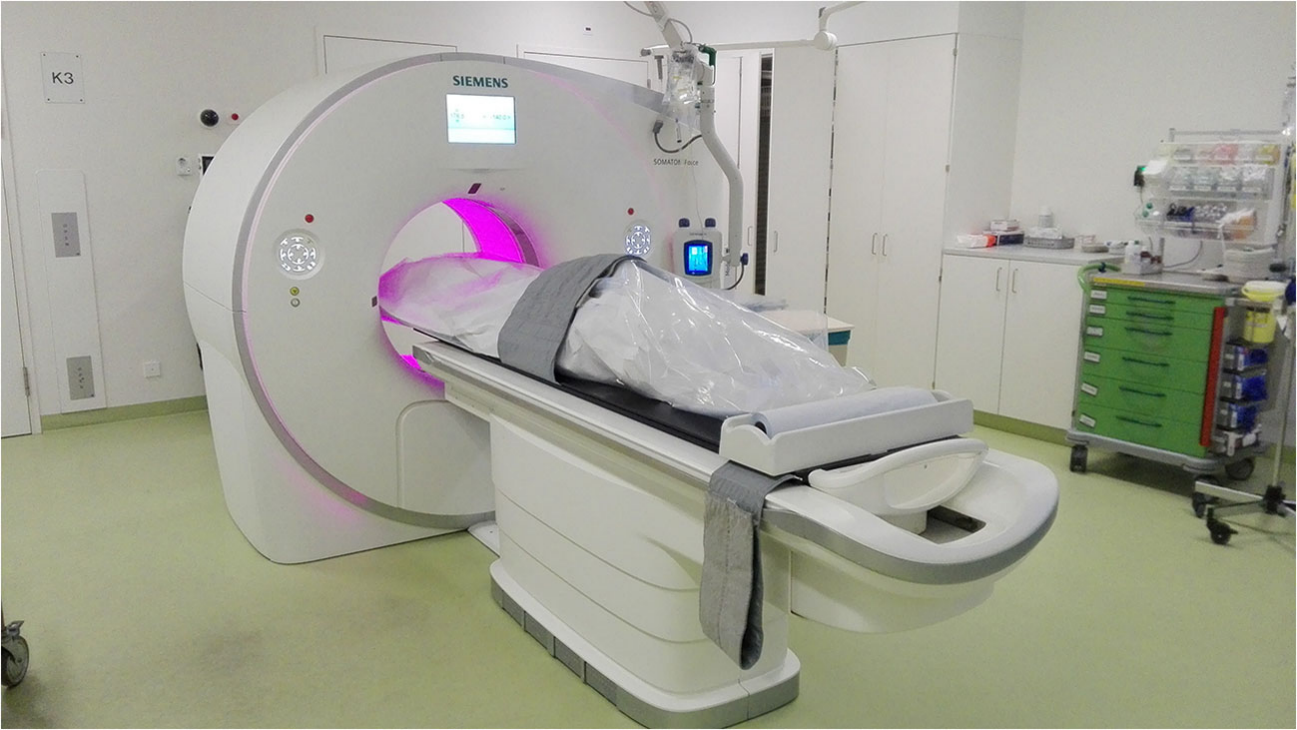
Scanning of study body in a body bag on the Siemens SOMATOM Force® Dual source CT scanner
